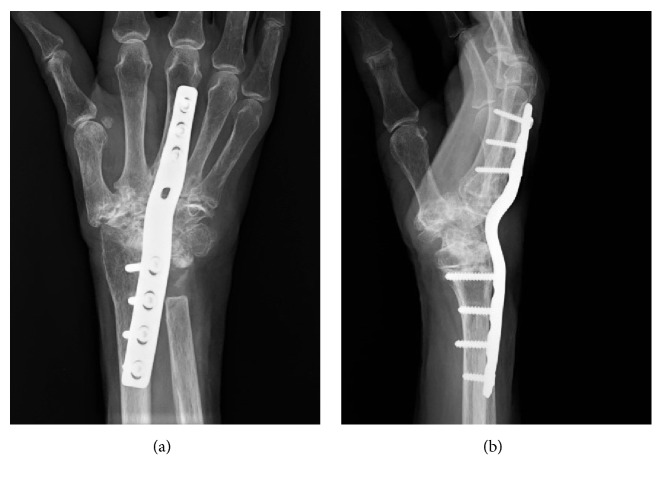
Representative anteroposterior (a) and lateral (b) radiographs of wrist arthrodesis using the AO wrist fusion plate.
